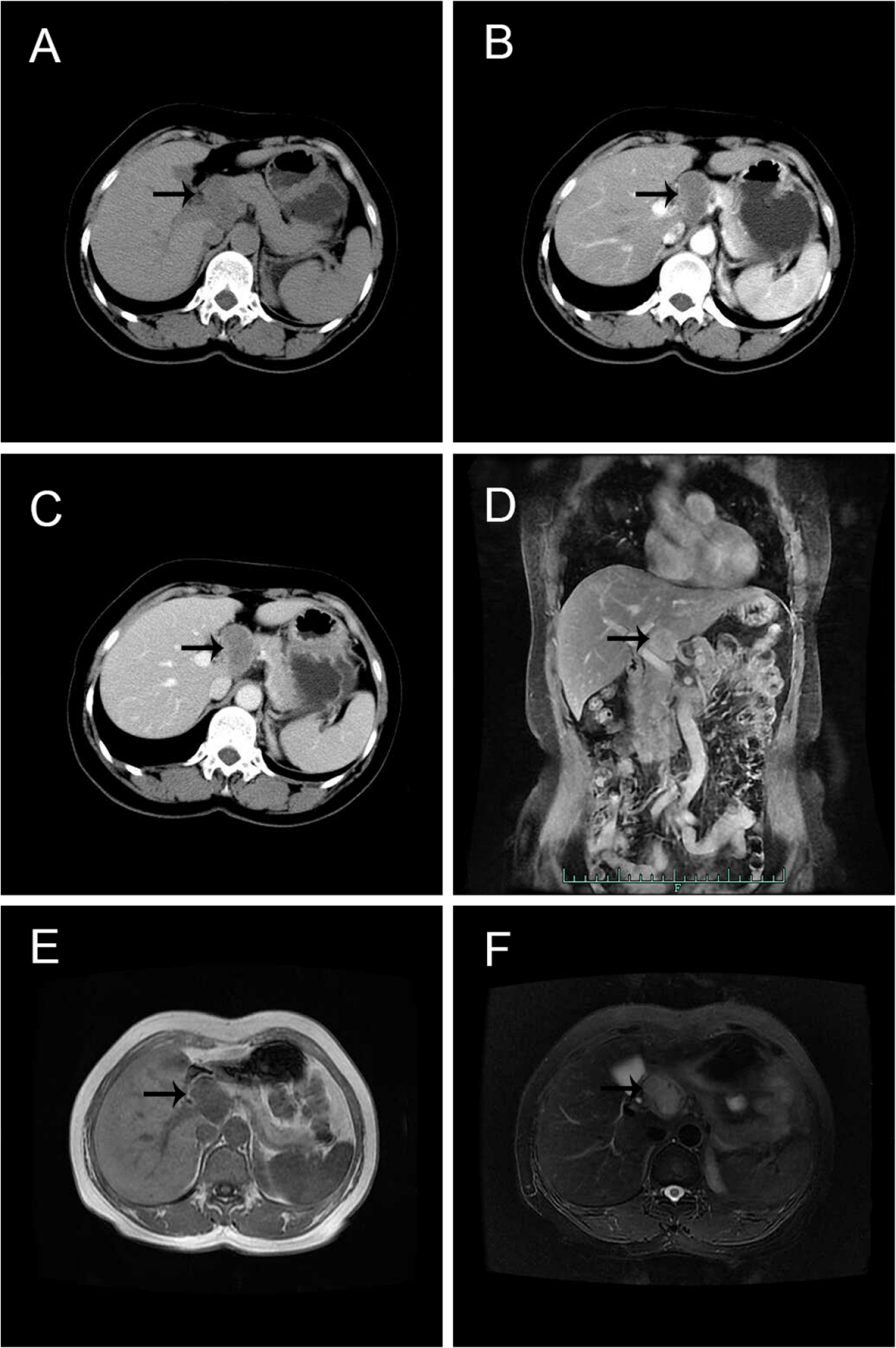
Abdominal CT and MRI findings of the patient. a Plain CT scan revealed a 4.0 × 3.2 × 3.0-cm hypodense mass in the porta hepatis (*black arrow*). b Enhanced CT scan revealed a mildly enhanced mass in arterial phase (*black arrow*). c Moderately enhanced mass in portal phase (*black arrow*). d MRI revealed a 4.0 × 3.0-cm mass in the sagittal section (*black arrow*). e Low signal intensity in T1-weighted imaging (*black arrow*). f High signal intensity in T2-weighted imaging (*black arrow*)
